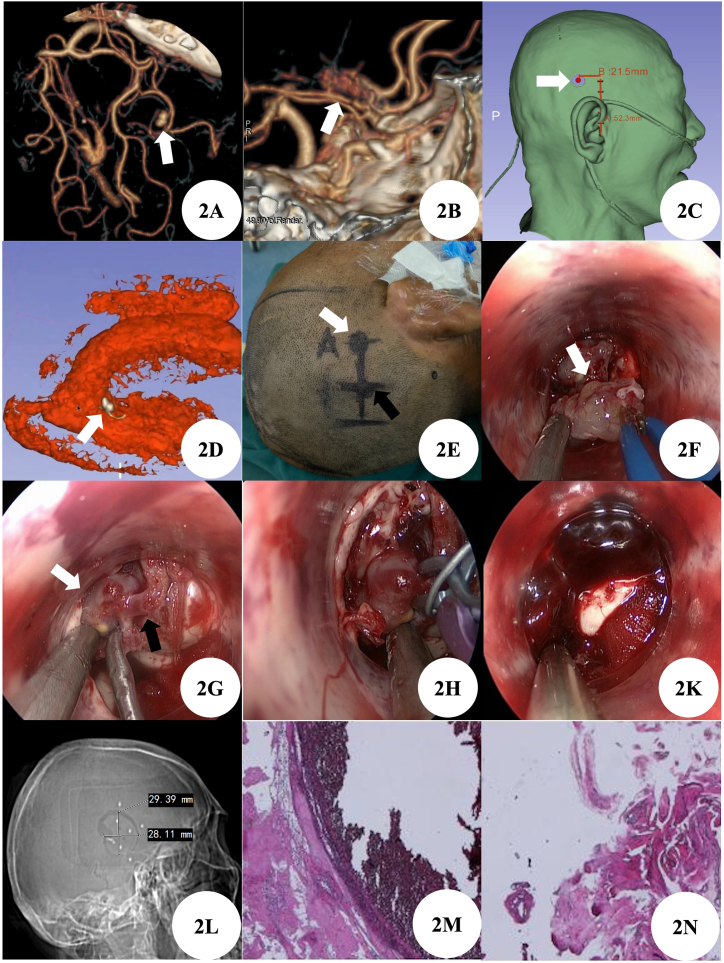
2A：Preoperative Brain CTA showed a distal segment aneurysm of the right anterior choroidal artery; 2B: Preoperative Brain CTA showed puff of smoke sign; 2C: 3D slicer reconstruction of the aneurysm surface projection; 2D: Relationship reconstructed by 3D slicer between the aneurysm and ventricular system; 2E: According to the projection of the body surface, locate the aneurysm (white arrow) and design the surgical incision line (black arrow); 2F: Choroid plexus papilloma was seen under neuroendoscope during operation; 2G: Aneurysm (white arrow) and Parent artery (black arrow) were seen under neuroendoscope during operation; 2H: Clipping aneurysm by using neuroendoscopic; 2K: Removaling intraventricular hematoma by using neuroendoscopic; 2L: Postoperative Brain CT showed that the size of bone window was about 3cm; 2 M: Pathological examination of postoperative aneurysm; 2 N: Pathological examination of choroid plexus papilloma after operation.
